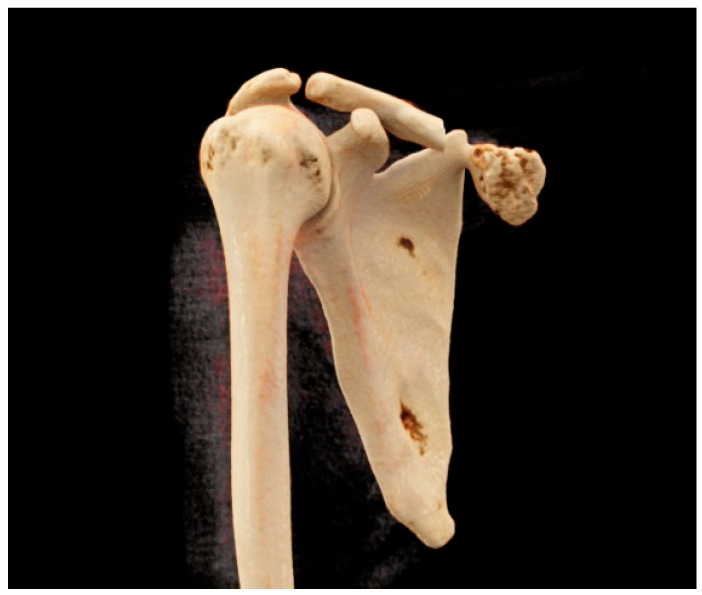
Erosion of the scapular bone (computed tomography scan).
